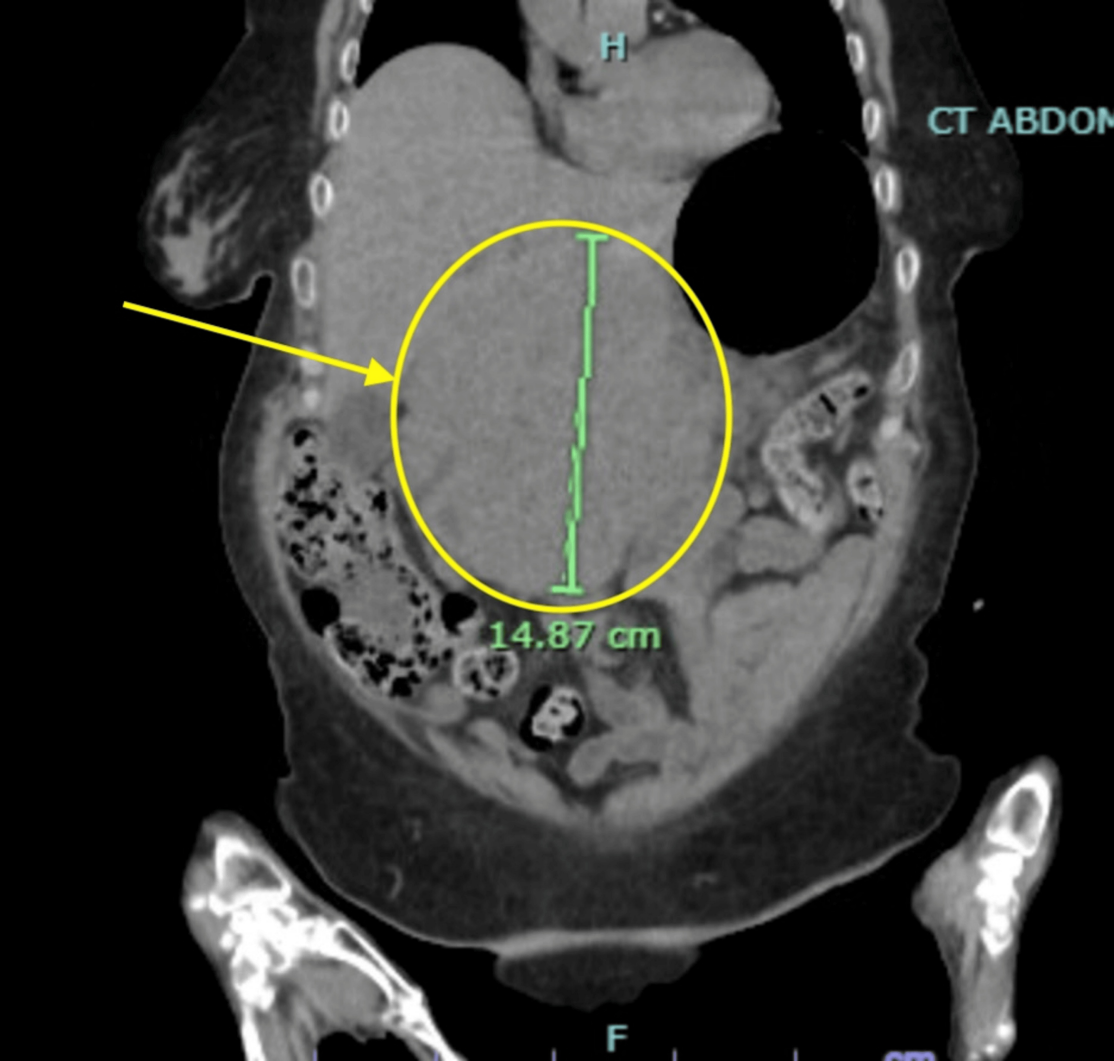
Coronal view of the initial CT of the chest, abdomen, and pelvis. The image displays a coronal cross-section from the patient’s CT scan of the chest, abdomen, and pelvis, obtained in the emergency department due to elevated liver function tests (see Table 2). The yellow circle and arrow indicate a large abdominal mass measuring a length of 14.87 cm. This is a different view of the same CT scan and the same mass indicated in Figure 1.
